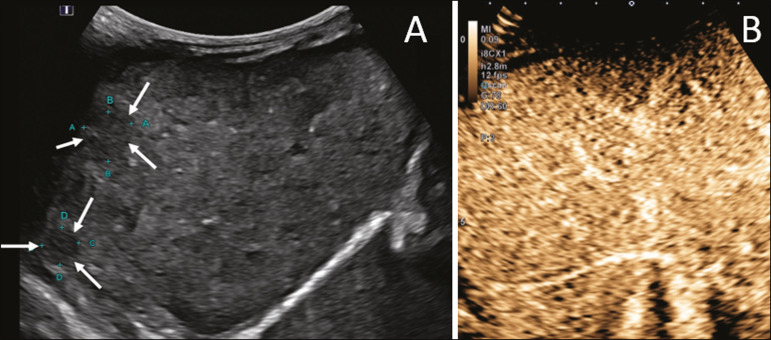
A 12-year-old patent with chronic liver disease and multiple nodules on ultrasound. A: B-mode ultrasound showing multiple hypoechoic nodules (arrows). B: On contrast-enhanced ultrasound, note that the enhancement of the nodules is similar to that of the adjacent liver parenchyma, on the arterial, portal venous, and delayed-phase images, without washout, consistent with regenerative nodules.
